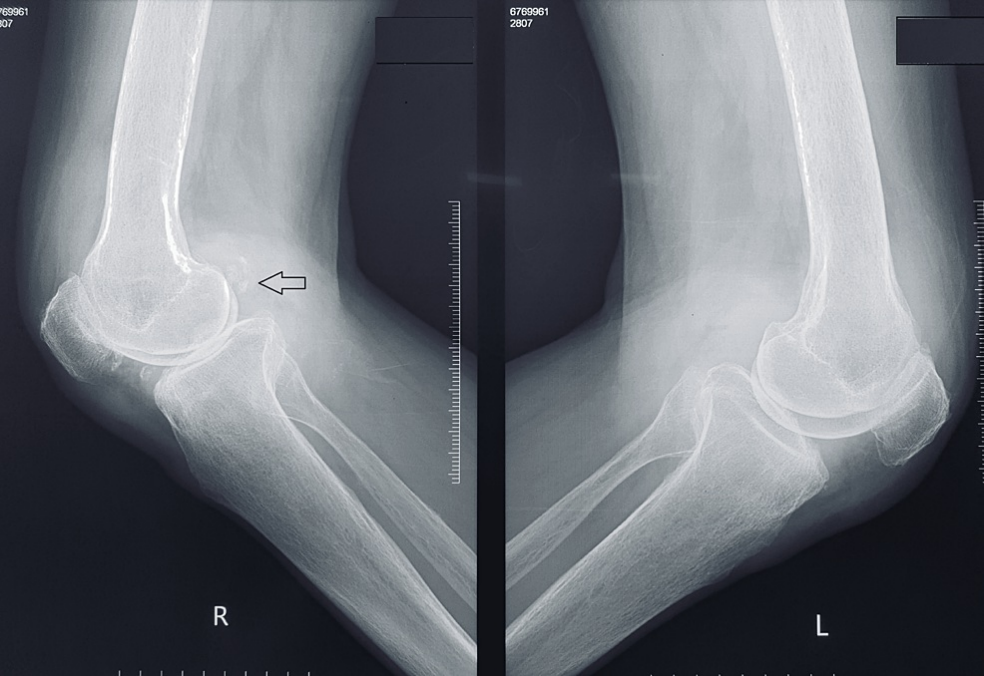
Lateral view plain radiography of both knee joints suggesting features of arthritis.
